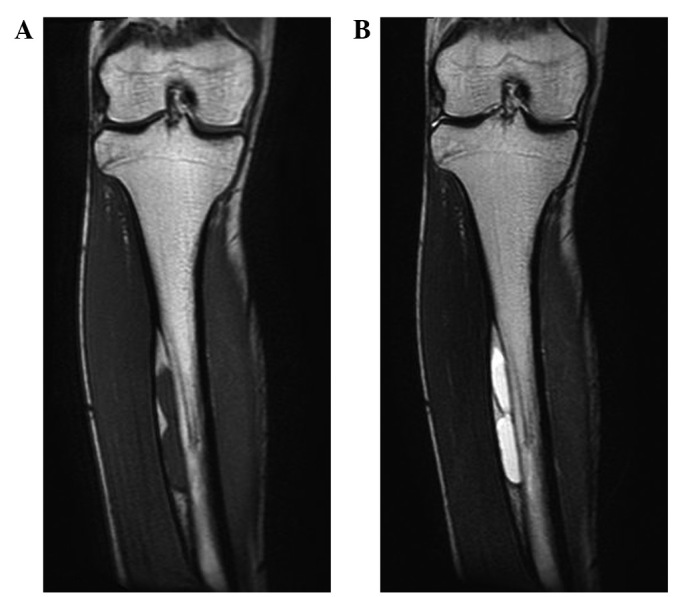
(A) Coronal T1-weighted MRI image revealing low-signal intensity in the central portion and high-signal intensity in the peripheral portion. (B) Coronal T2-weighted MRI image exhibiting high-signal intensity in the central and peripheral portions. MRI, magnetic resonance imaging.
